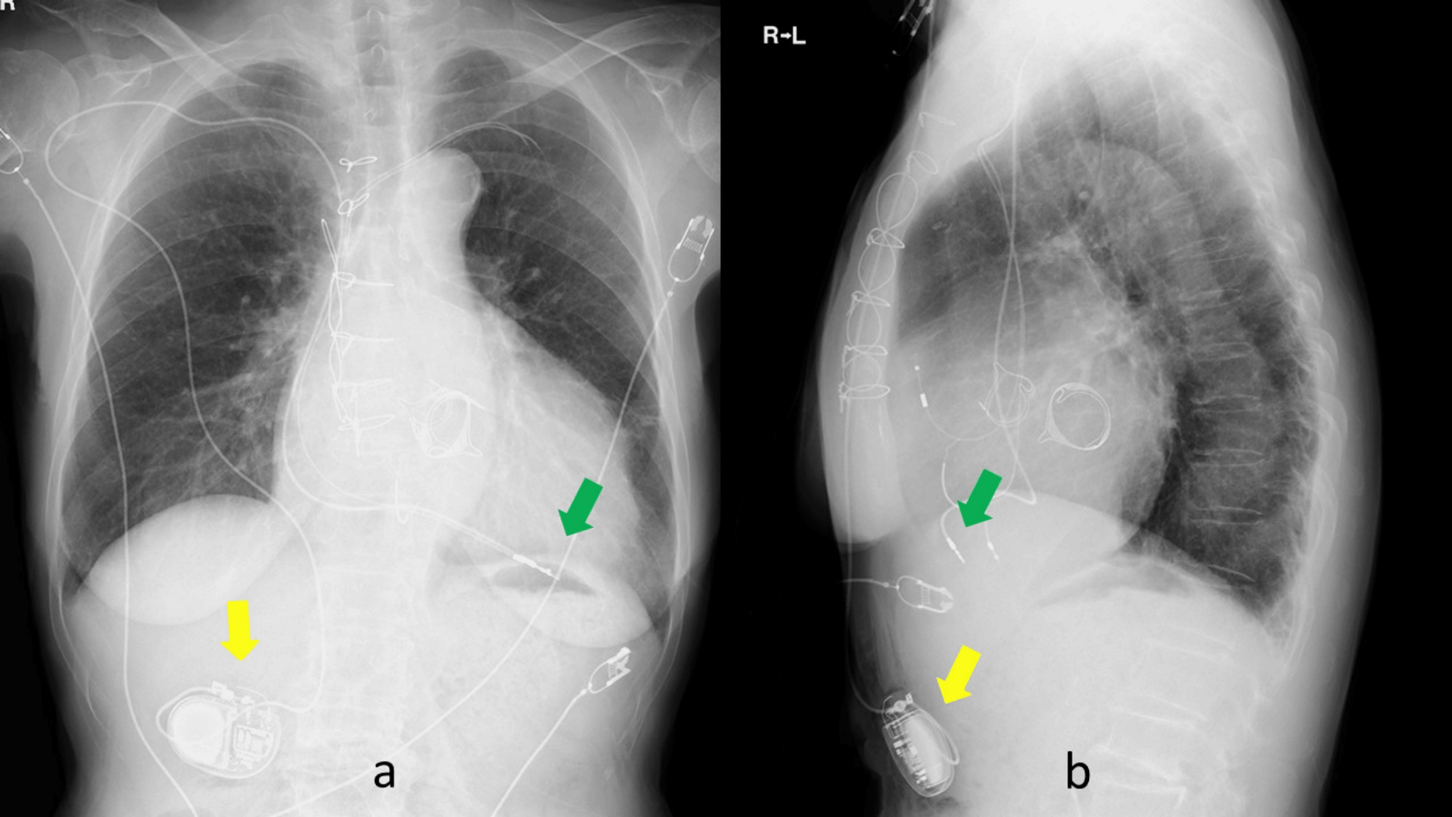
Chest X-ray films. Yellow arrows indicate the relocated pacemaker in the abdomen. Green arrows indicate the tip of the pacing lead at the right ventricle. (a) Frontal and (b) lateral.
